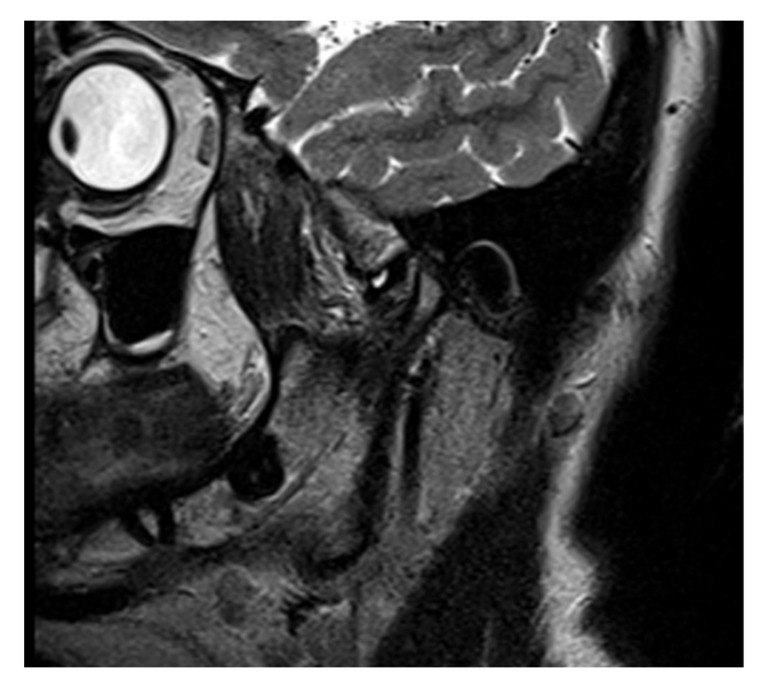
Sagittal oblique closed mouth position T2-weighted MRI of the left joint: joint effusion.
